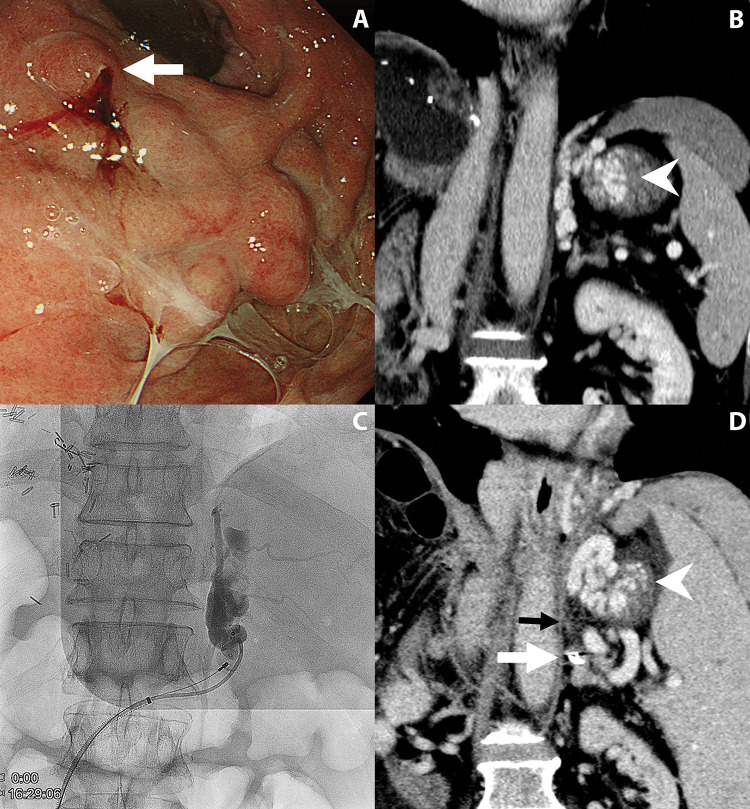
A 62-year-old man who underwent plug-assisted retrograde transvenous obliteration. A. Endoscopic examination shows gastric variceal bleeding (arrow). B. Coronal contrast-enhanced CT scan reveals a large and tortuous varix (arrowhead) at the gastric fundus. C. Fluoroscopic image shows a vascular plug deployed at the gastrorenal shunt. After injection of gelatin sponge slurry, gastric varices are only partially opacified. Selective catheterization of collaterals failed due to severe tortuosity. D. Follow-up CT scan shows an obliterated gastrorenal shunt (black arrow) with a vascular plug (white arrow) and residual gastric varices (arrowhead).
